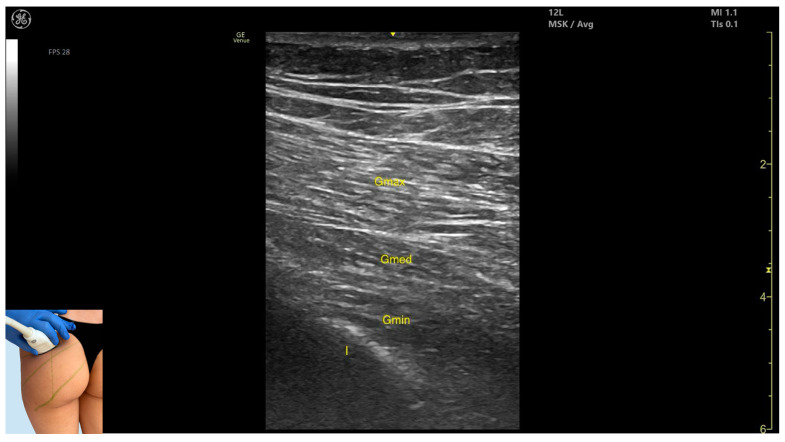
Ultrasound anatomy of *gluteus maximus* (Gmax) with key landmarks: Gmax—*gluteus maximus*; Gmed—*gluteus medius*; Gmin—*gluteus minimus*; I—iliac bone.
